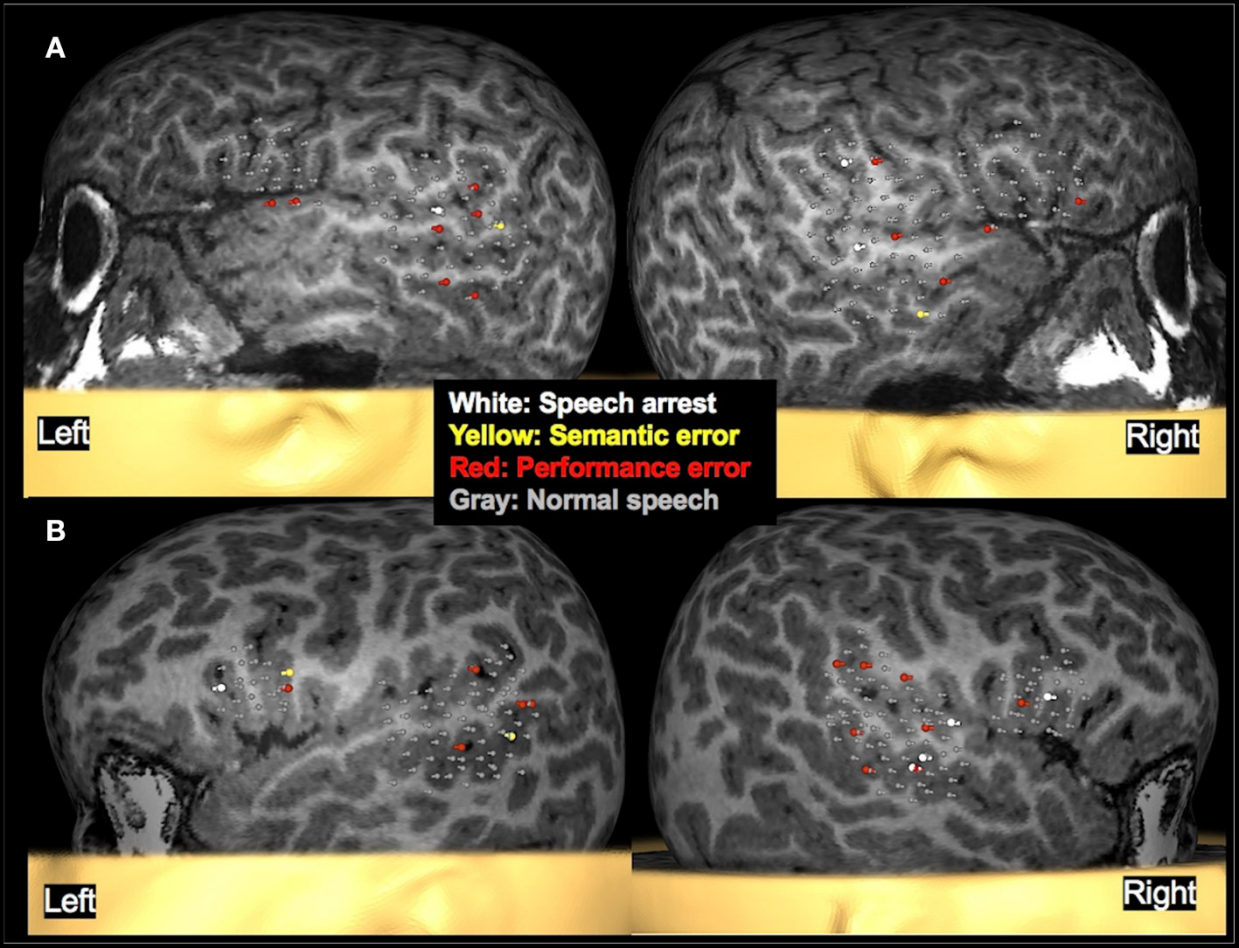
Examples of language mapping with TMS. Speech errors in the form of speech arrest, semantic errors, and performance errors were elicited in both hemispheres. (A): Language mapping with TMS in a 5.6-year-old female with refractory cryptogenic focal epilepsy and asymptomatic cervical and thoracic syringohydromyelia. Her brain MRI was normal. TMS language mapping was completed using a color naming task and showed bilateral dominance for expressive language. (B): Language mapping with TMS in a 5.6-year-old male with right parietal cortical dysplasia that was in the inferior parietal lobule, predominantly superior to the marginal gyrus. TMS language mapping was completed using an object naming task and indicated a right hemisphere dominance for expressive language.
